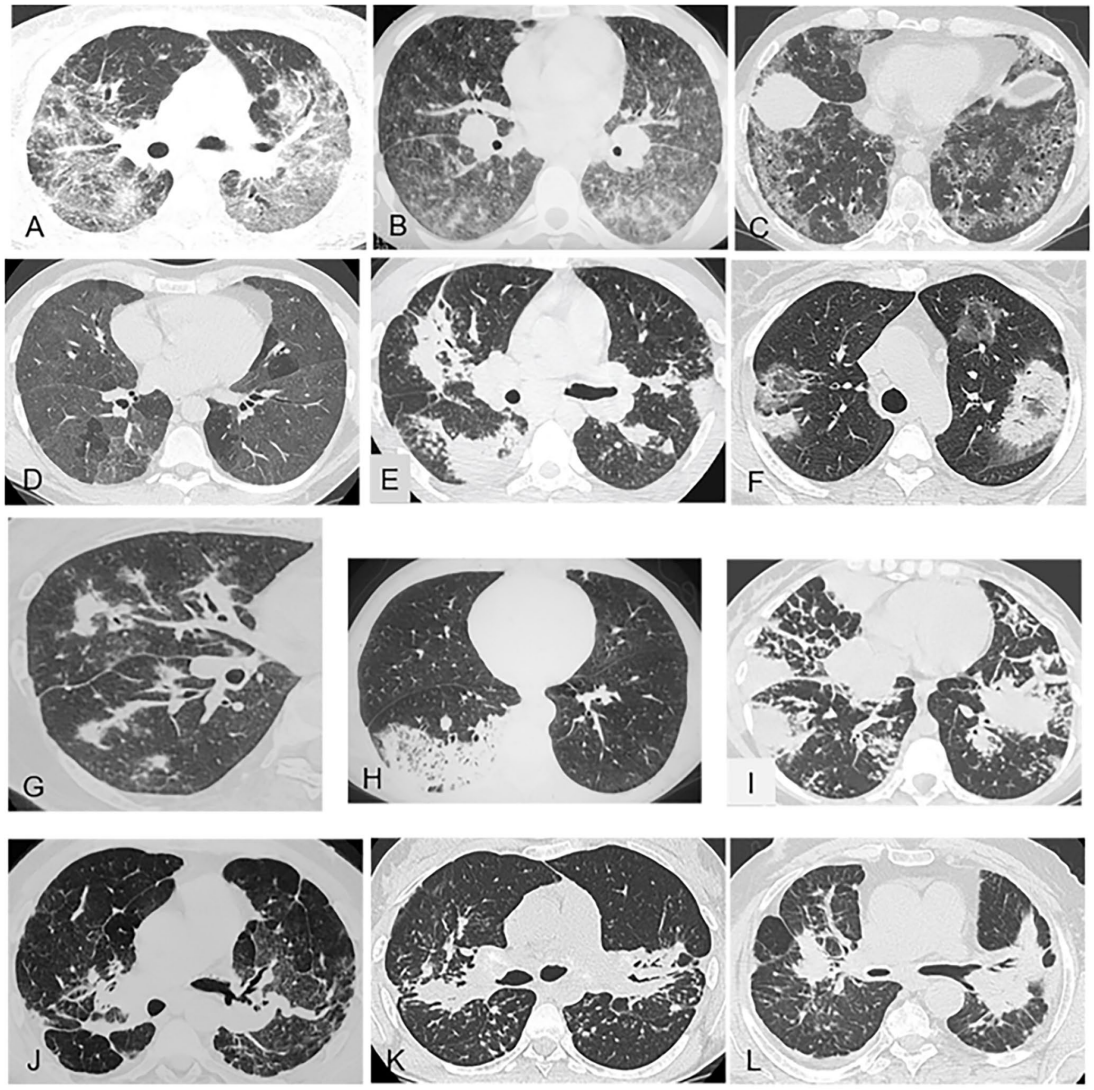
Representative of gound-glass opacities (A–D) and alveolar consolidations (E–I) and masses (J–L) on chest CT (pulmonary window). (A) Pulmonary sarcoidosis with predominant, diffuse, ground-glass opacities combined with lymphatic micronodules. (B) Chronic beryllium disease: heterogeneous ground-glass opacities with bilateral hilar and mediastinal lymphadenopathy. (C) Nonspecific interstitial pneumonia: diffuse ground-glass opacities and fine reticulations sparing the subpleural lung. (D) Hypersensitivity pneumonitis: diffuse ground-glass opacities and multiple clear lobules trapped in expiration. (E) Pulmonary sarcoidosis with bilateral alveolar consolidations and lymphatic micronodules. (F) Cryptogenic organizing pneumonia: bilateral peripheral consolidations with lower-density (ground-glass) centers. (G) Granulomatosis with polyangiitis: alveolar consolidation related to a pulmonary vessel. (H) Lepidic-growth carcinoma: pneumonic form with a unilateral focus. (I) Lymphoma: bilateral alveolar consolidations with a limited number of septal reticulations and micronodules. (J) Fibrosing pulmonary sarcoidosis: typical presentation with bilateral hilar masses and bronchial distortion. (K) Sarcoidosis with bilateral masses in the posterior segments of the upper lobes and micronodules. (L) Coal miner’s pneumoconiosis: bilateral mass-like lesions in the upper lobes.
